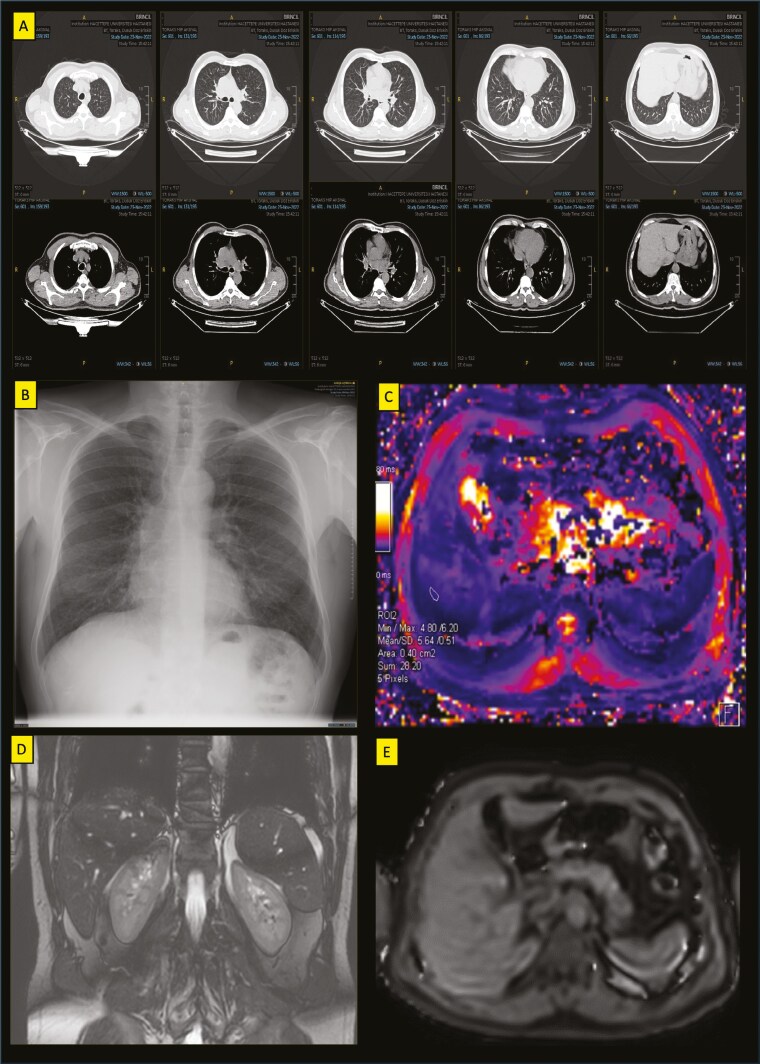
Various sections of thorax CT findings (A), Posteroanterior chest radiography (B), Thalassemia iron accumulation MRI (Liver Section) Liver T2* value on the T2 star map is approximately 5.6 ms and decreased in accordance with minimal iron accumulation (C), abdomen dynamic MRI, T2 liver and spleen are clearly black (hypointense) (D) T2* sequence, liver parenchyma T2* measurement value is 4.9 ms, compatible with significant iron accumulation (E).
